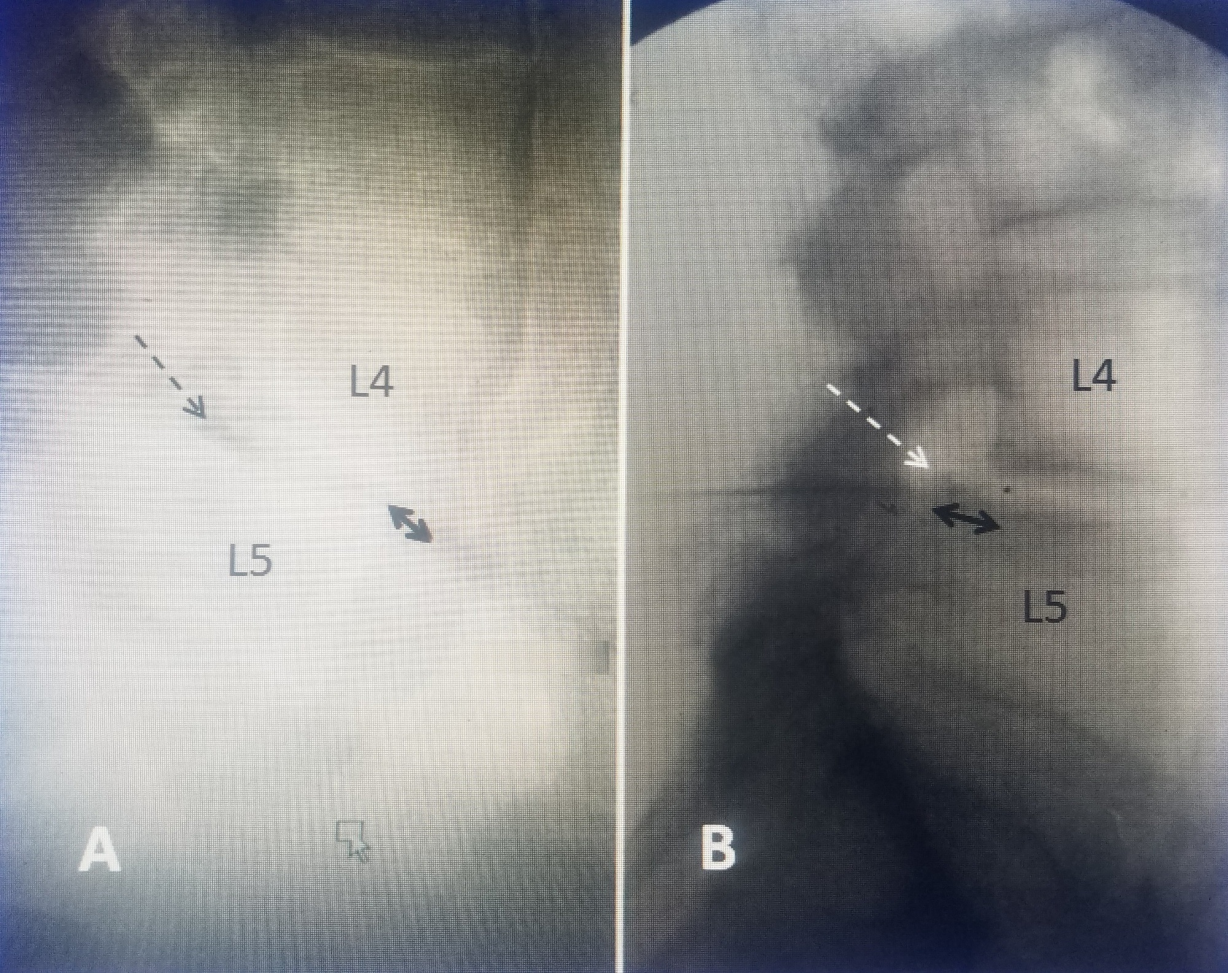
Comparison of different quality lateral views using plain X-rays and digital imaging to evaluate L4-5 spondylolisthesis in the same patient. A: Initial poor quality plain X-rays showing grade 1 spondylolisthesis at L4-5 which is better seen ventrally (double-headed black arrow). The neural foramina can barely be seen (dashed black arrow). B: Better digital radiographic images in the same patient clearly showing the neural foramina (dashed white arrow) as well as the grade 1 spondylolisthesis (double-headed black arrow).
